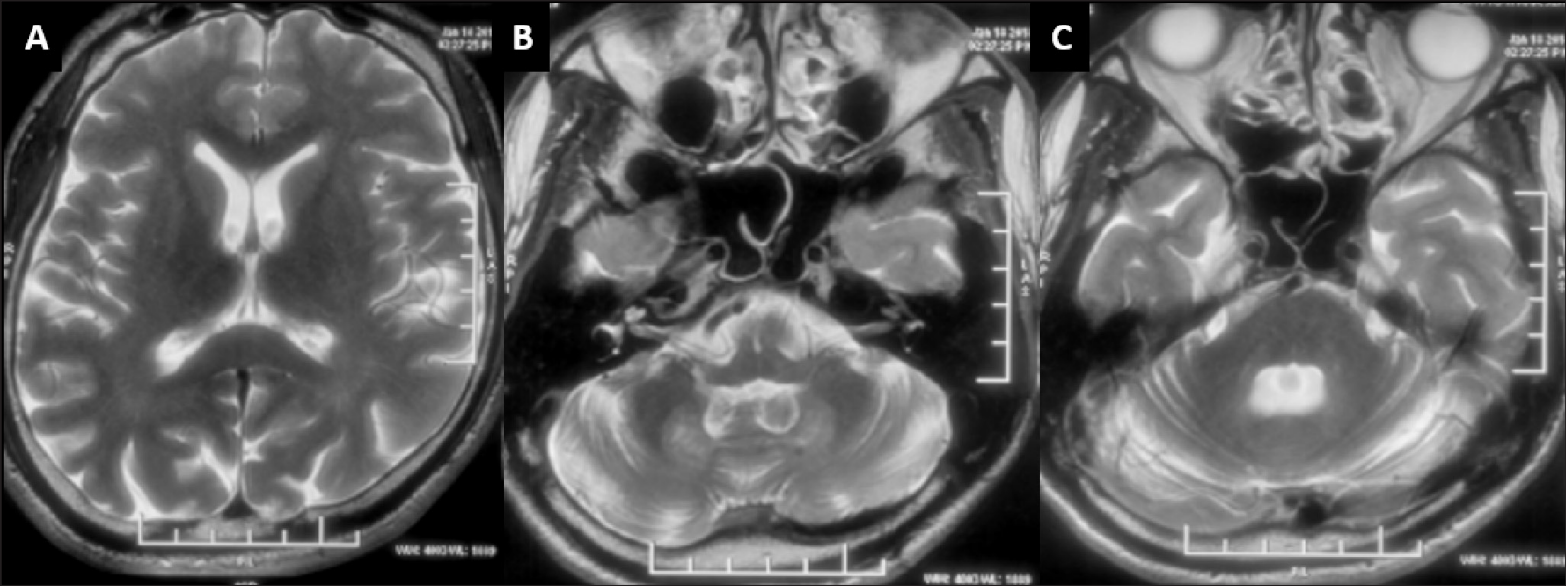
MRI of Patient-4. T2 axial images (A-C) showing mild diffuse cerebral and cerebellar atrophy with symmetric dentate nuclei hyperintensities.
